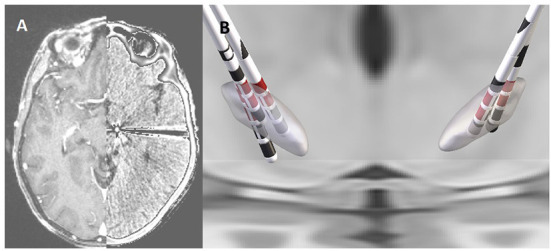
Co-registered pre-operative MRI and post-operative CT scan from an individual participant (A). Reconstruction of the DBS leads in the STN for each of the five participants with the stimulation contacts shown in red (B).
